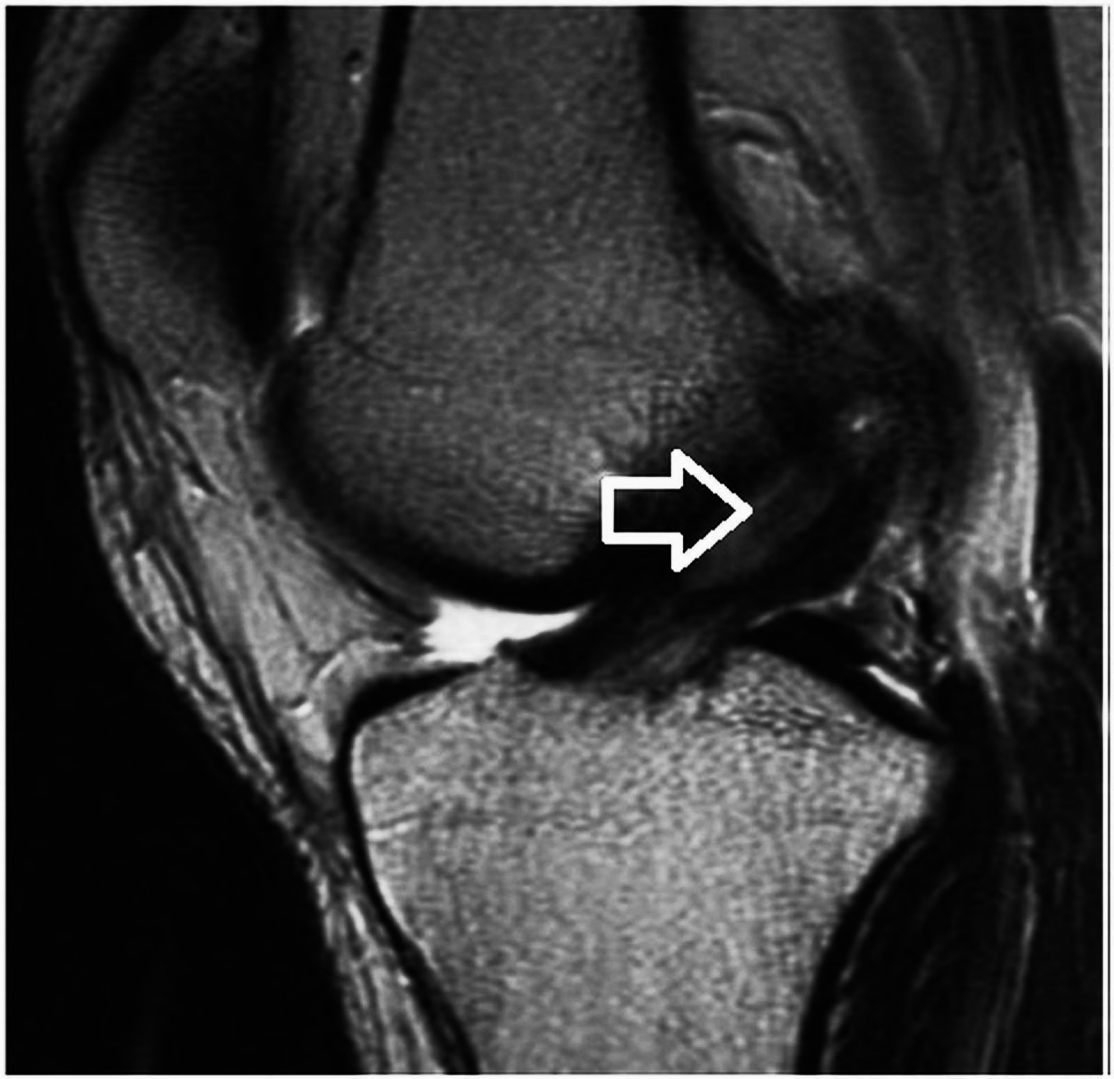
Sagittal T2-weighted magnetic resonance image, which shows intact ACL fibers with ganglion cyst (arrow). ACL: anterior cruciate ligament
